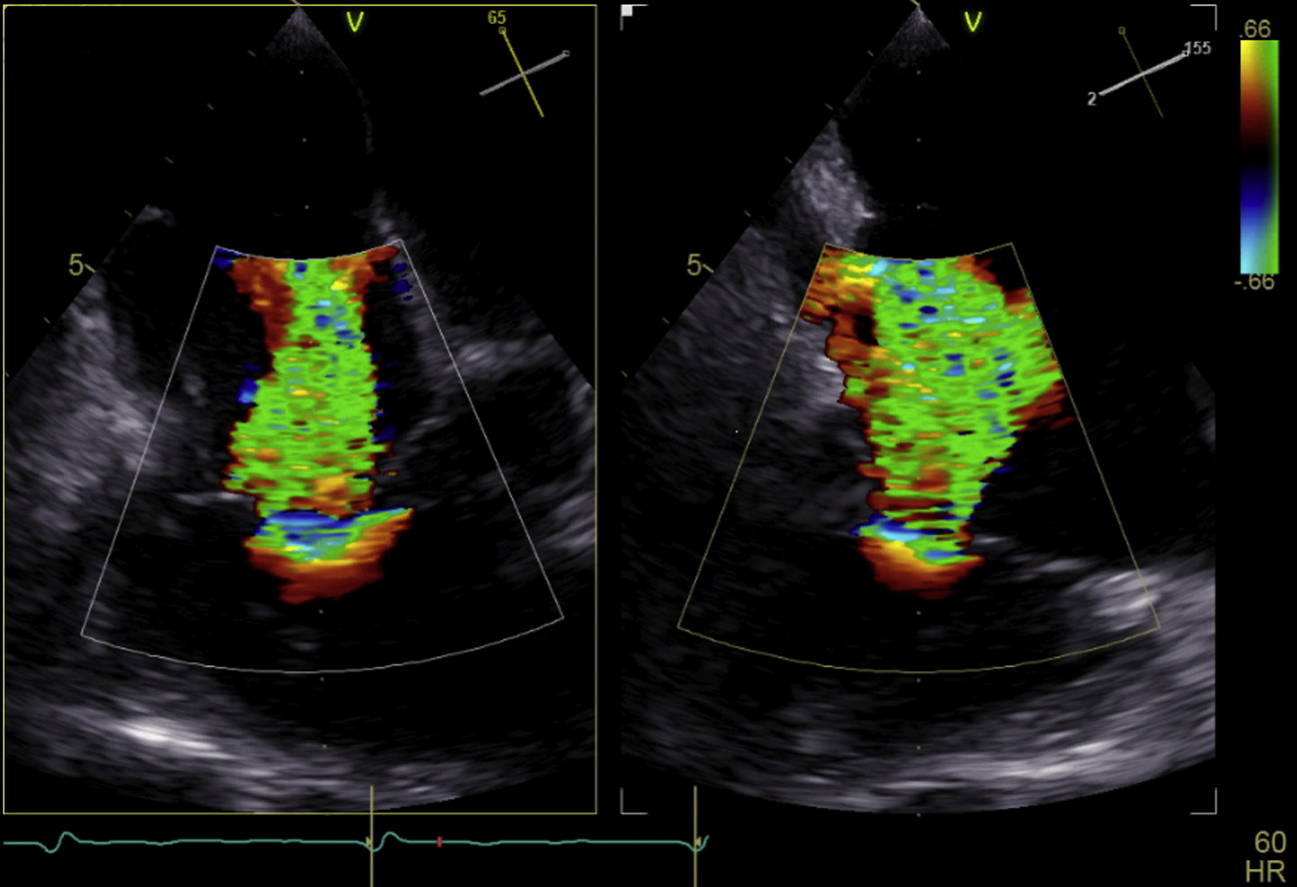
Biplane Echocardiography Showing Massive Tricuspid Regurgitation
